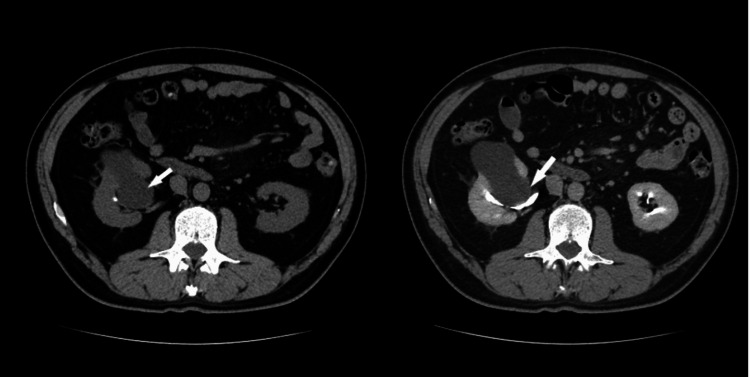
Non-enhanced CT scan (NECT) (a) and contrast-enhanced CT (CECT) scan during the urographic phase (b), a notable observation is evident. Specifically, there is the identification of a right parapelvic cyst, marked by the arrow, which protrudes into the renal sinus from the adjacent tissue. Despite its presence, there is no observed compression or pressure exerted upon the pelvicalyceal system. This figure is the original work of the authors. Patient consent for the use of the image was obtained, as mentioned in the patient consent form (point no. 12).
